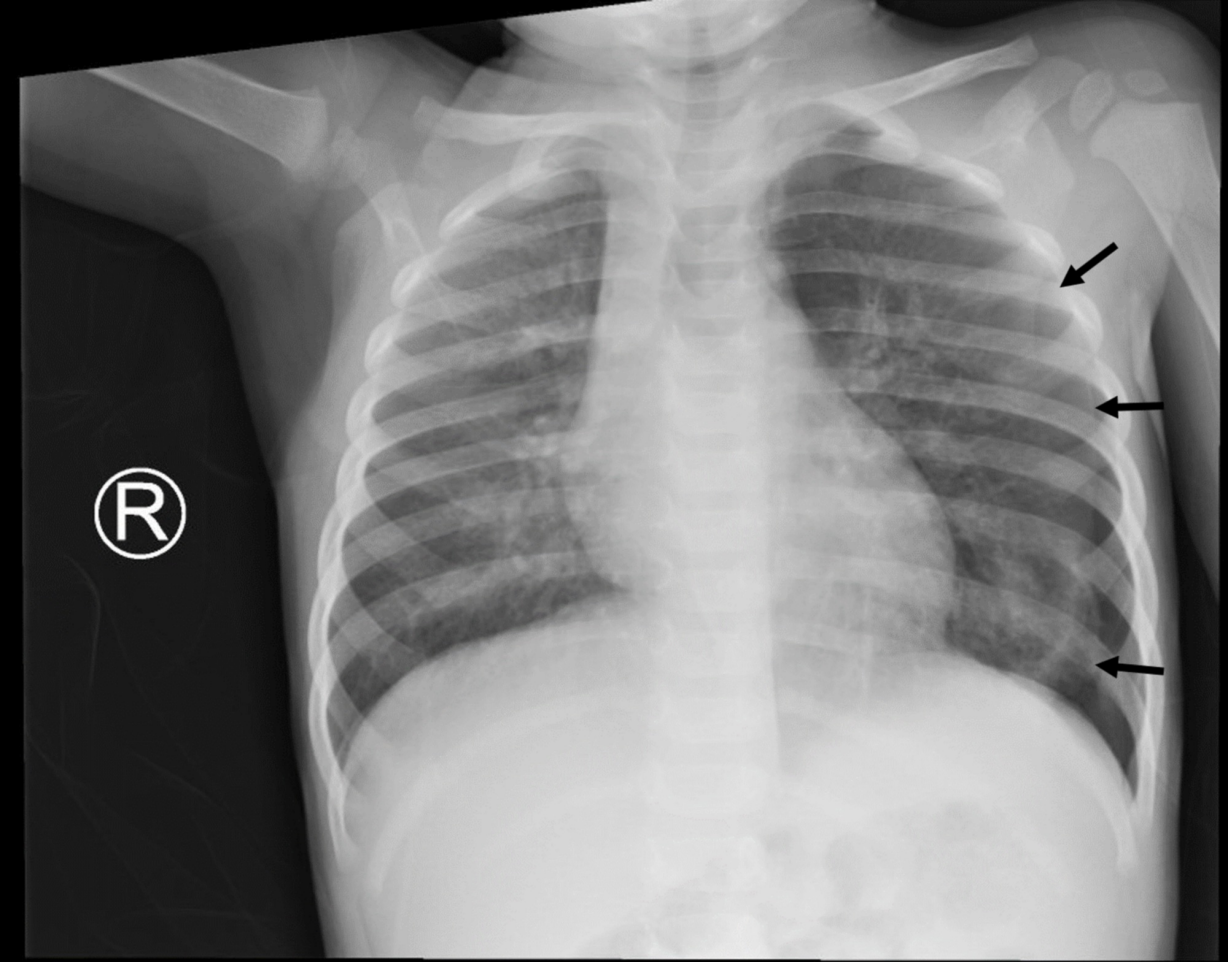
Chest X-ray on day four demonstrated left-sided pneumothorax on arrival from outside facility; black arrows outline the lateral aspect of the lung
